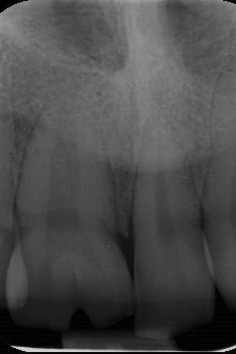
Radiographic image of the geminated right maxillary central showed a labial notch, leading to a partially bifid crown.
